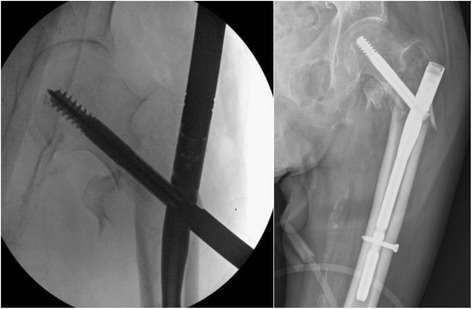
Radiographs of Zimmer nail with cephalic screw with TAD between 25 and 30 mm during surgery and after 5 months with mobilisation of the cephalic screw for a fracture 31-A2
